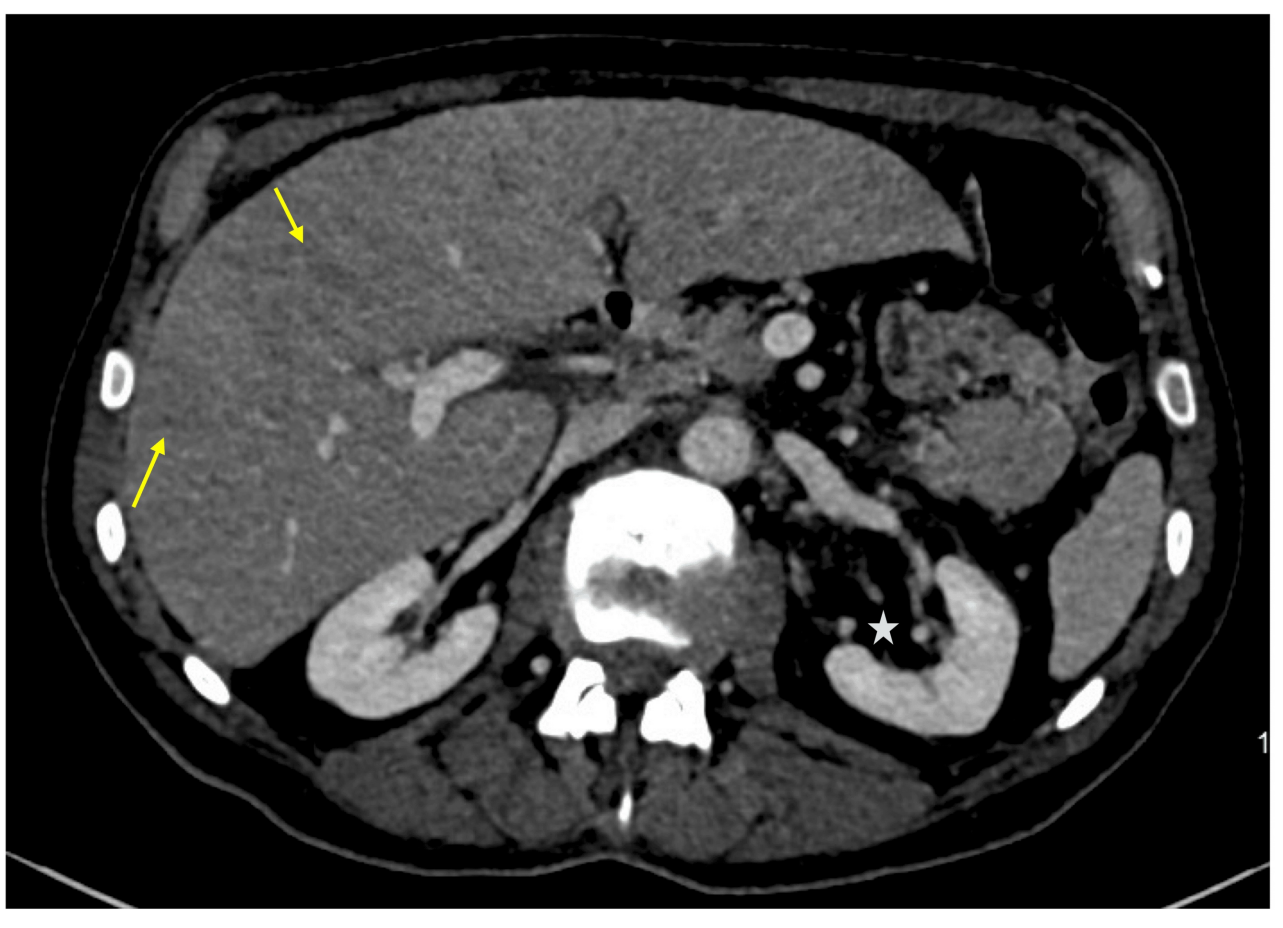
Axial computed tomography (CT) image of the thorax, abdomen, and pelvis showing slight heterogeneity of the liver The arrows point to heterogeneous liver enhancement. The star indicates left-sided retroperitoneal fat stranding.
